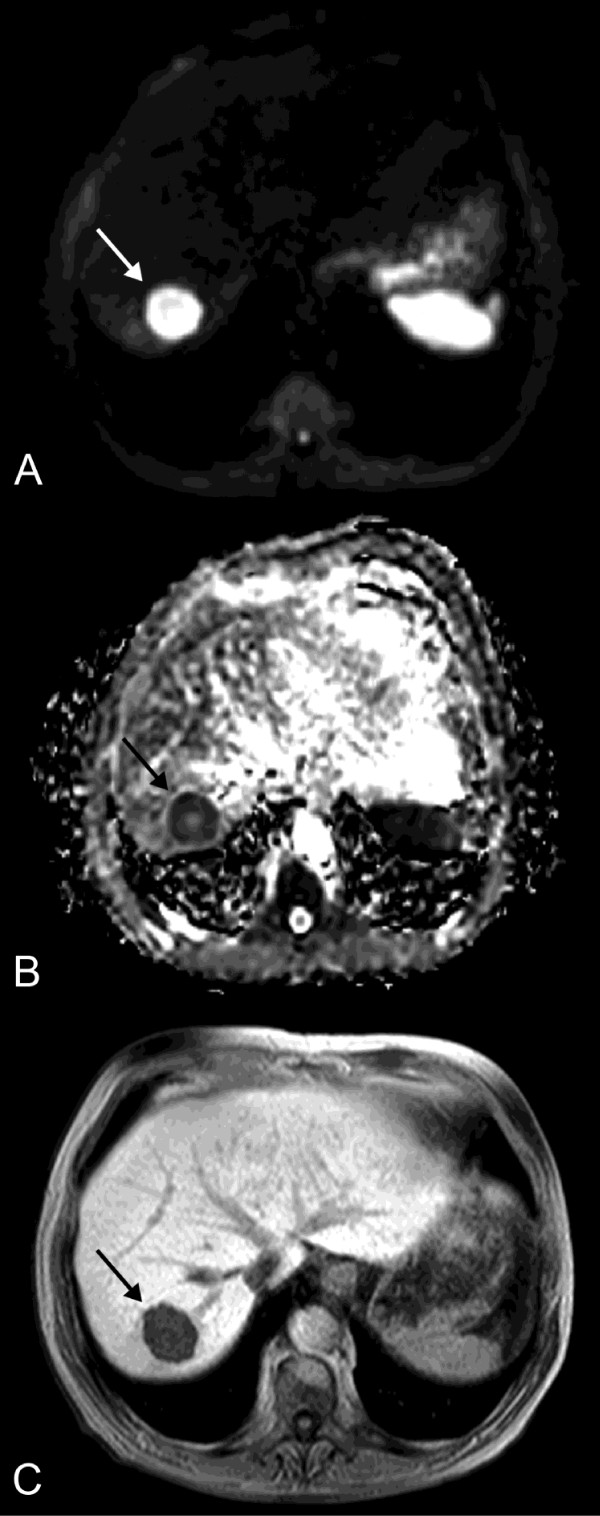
Baseline MRI preceding HDR-BT. Pre-treatment diffusion-weighted image (DWI) with *b *= 500 s/mm^2 ^(A), corresponding apparent diffusion coefficient (ADC) map (B) and T1w Gd-EOB-DTPA enhanced MR image in hepatocyte-selective (hepatobiliary) phase (C) of the same patient as in Figure 1 depict the colorectal metastasis in liver segment VII with a mean ADC of 1.29 × 10^-3 ^mm^2^s^-1 ^and a mean volume of 23.3 cm^3 ^(arrow).
